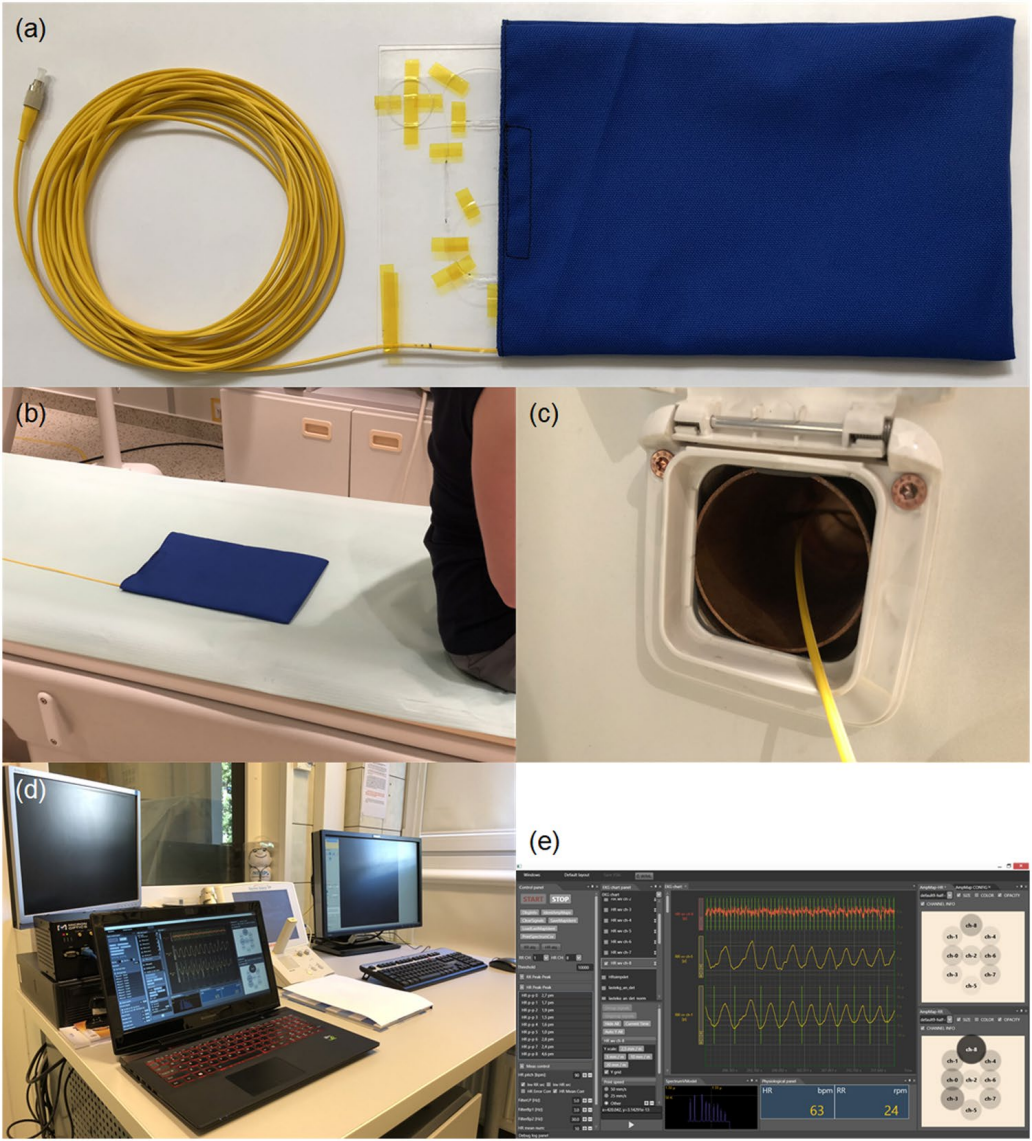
Main elements of the vital signs sensor system: the (a) sensor mat with partially exposed spring-board and an FBG element, (b) sensor mat on the mattress of the MRI track table, (c) optical fibre threaded through the wall opening, (d) interrogation module and the laptop PC in the operator room, and (e) main window of the application for signal visualisation, RR determination and data archiving.
